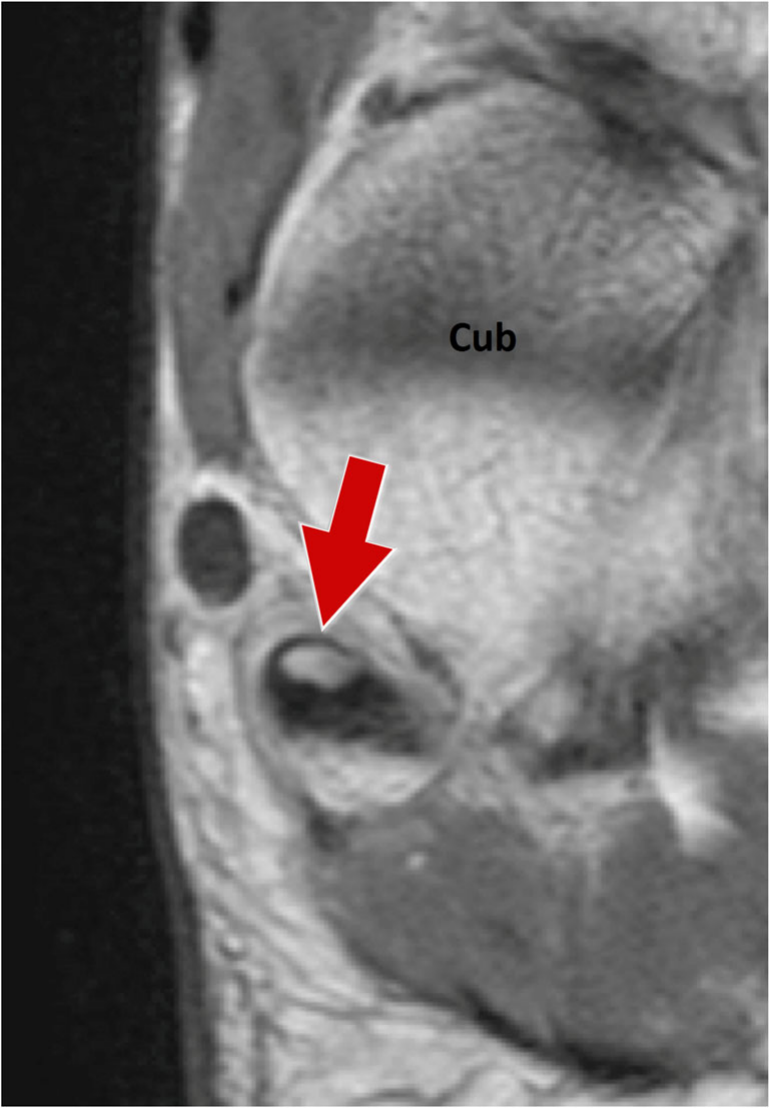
Normal os peroneum – magnetic resonance imaging. Coronal T1w plane. MRI allows optimal evaluation of the OP (*arrow*) bone marrow, which shows a signal similar to that of the adjacent cuboid and also correctly depicts pathologies of the synovial sheaths. *Cub* cuboid
